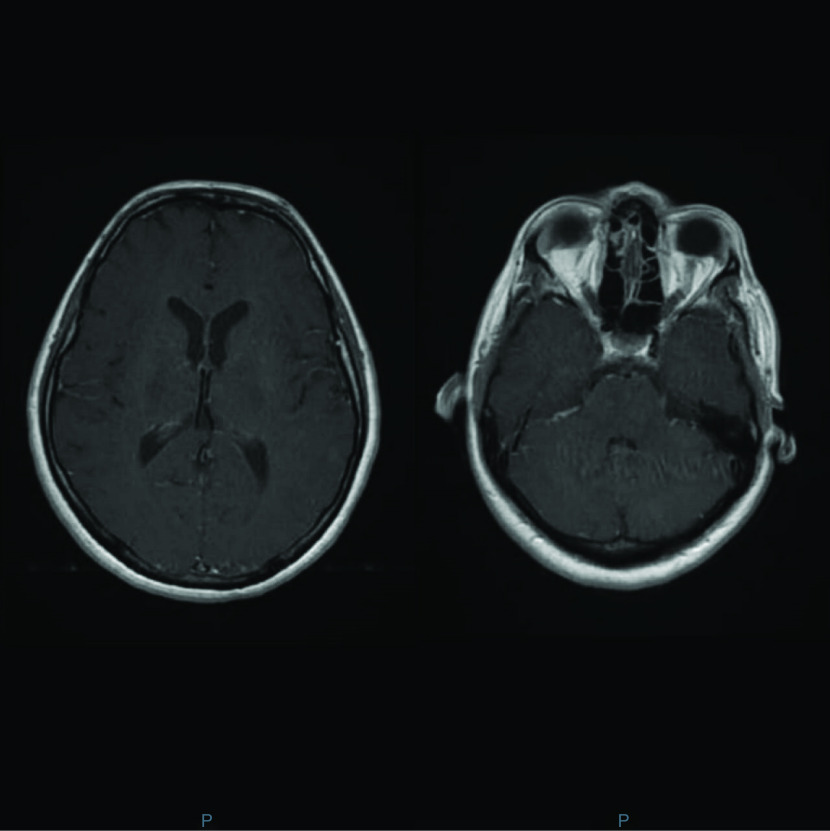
Similar imaging findings on brain MRI were noted 4 days later.
